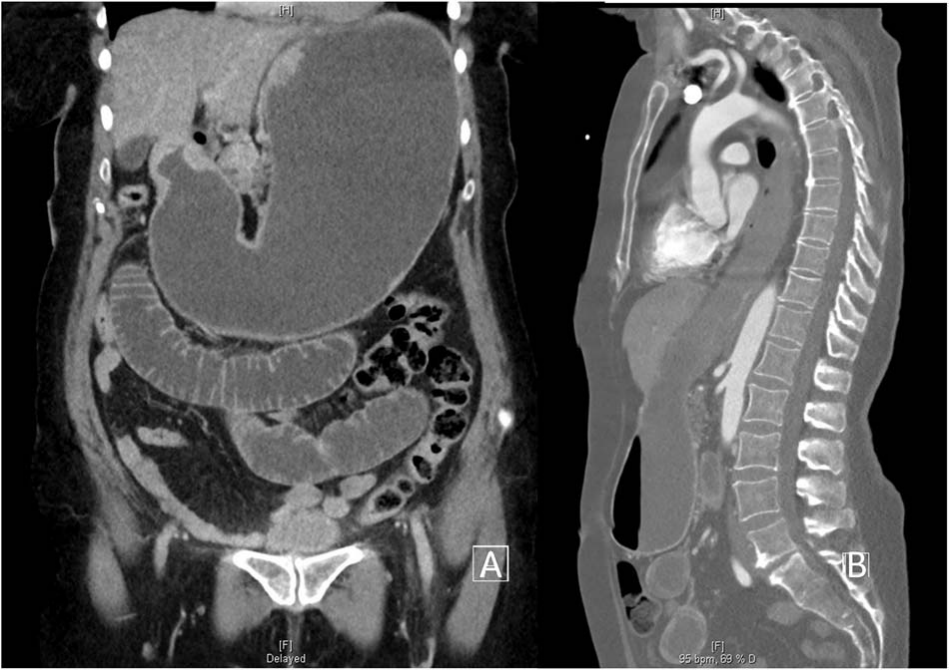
(A) Coronal CT cross-section demonstrating gross bowel and stomach distension. (B) Sagittal CT cross-section demonstrating obstruction and reflux of stomach contents into oesophagus.
